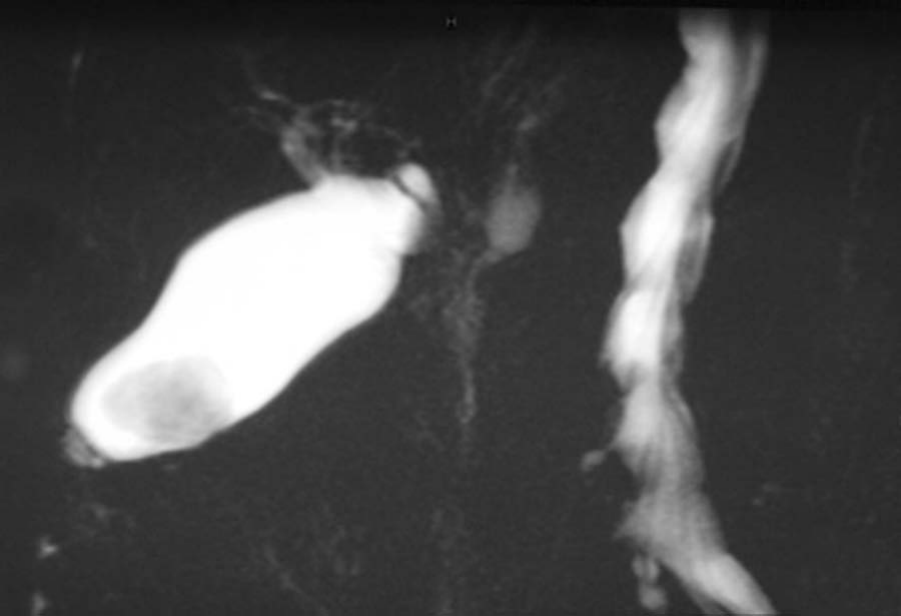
Case 2. Three-dimensional reconstruction of axial, T2-weighted magnetic resonance imaging revealing a solid structure (arrow) adhering to the inner gallbladder surface, surrounded by bile.
